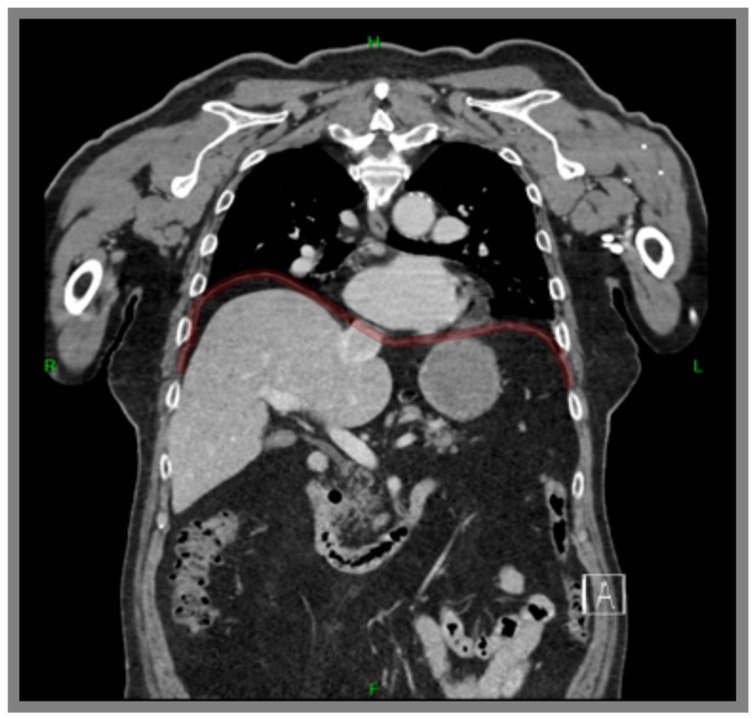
Computer tomography (CT) image of the studied patient with right hemidiaphragm paralysis (the contour is highlighted by a red line). On the right side, the paralyzed hemidiaphragm is substantially elevated in the thorax, resulting in spatial limitation for the lung to expand and relocation of the liver and the heart.
